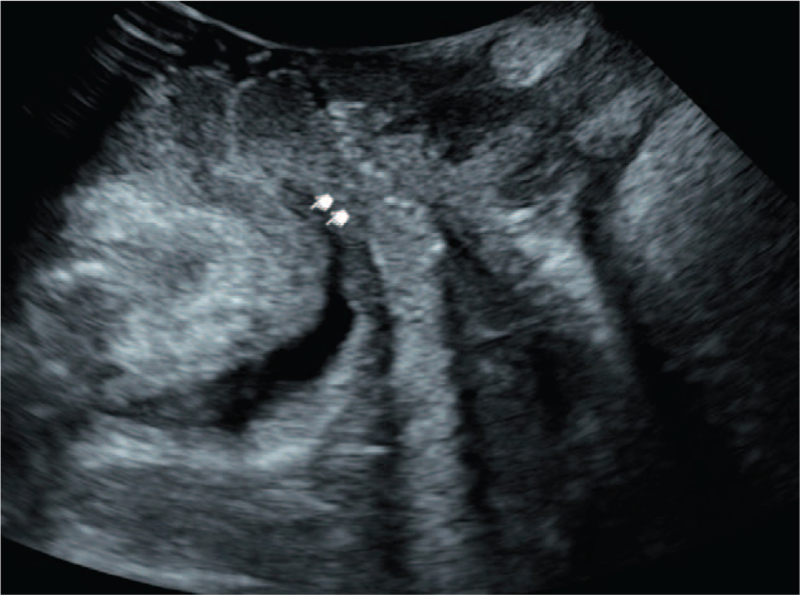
Ultrasonic image taken in the state of Valsalva maneuver.
